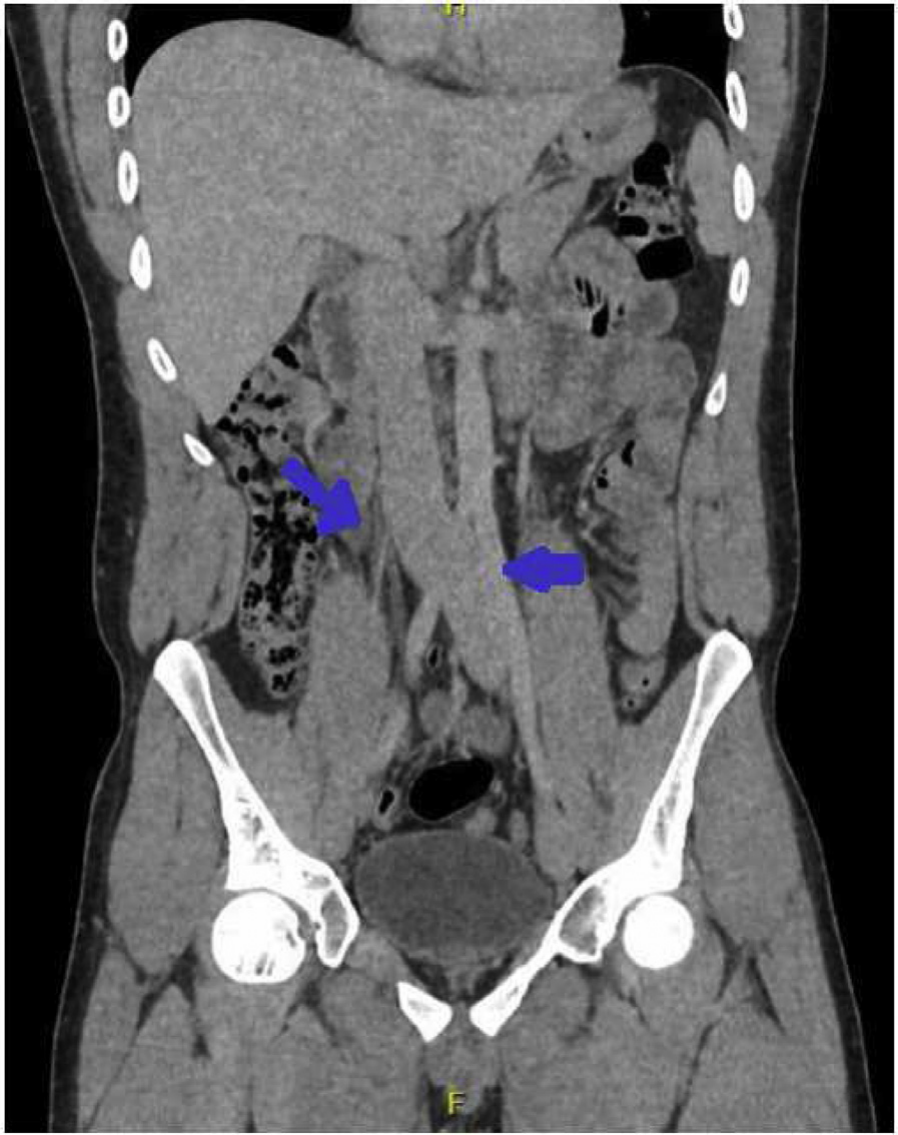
Coronal projection of computed tomography venogram (CTV) of abdomen and pelvis demonstrating absence of the right common iliac vein and a slightly dilated left common iliac vein.
